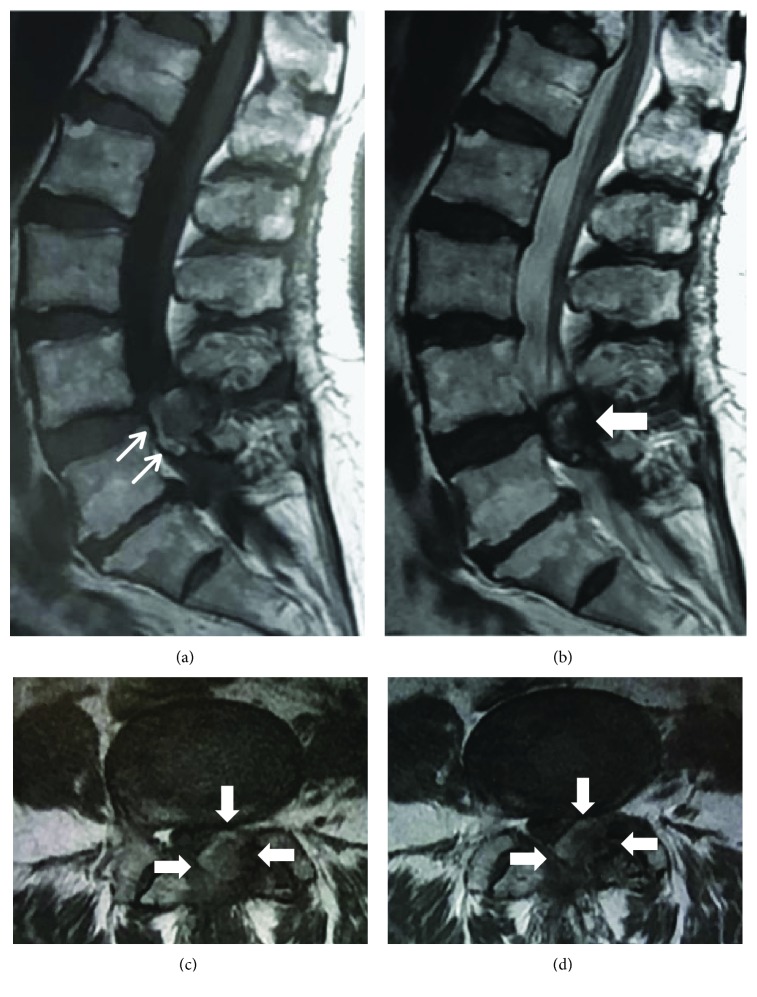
2^nd^ magnetic resonance images (MRI) showing left posterior mass at the same area of the 1^st^ MRI. (a) Sagittal T1-weighted axial MRI reveals that there was a newly isointense mass present within the anterior part of the previously identified lesion. (b) Sagittal T2-weighted axial MRI reveals that the intensity inside the mass was a little extended. (c, d) T1-weighted axial MRI at L4/5 reveals the well-defined extradural mass in the left posterior aspect of the thecal sac. (c) There was no significant enhancement with Gd-based contrast (d).
